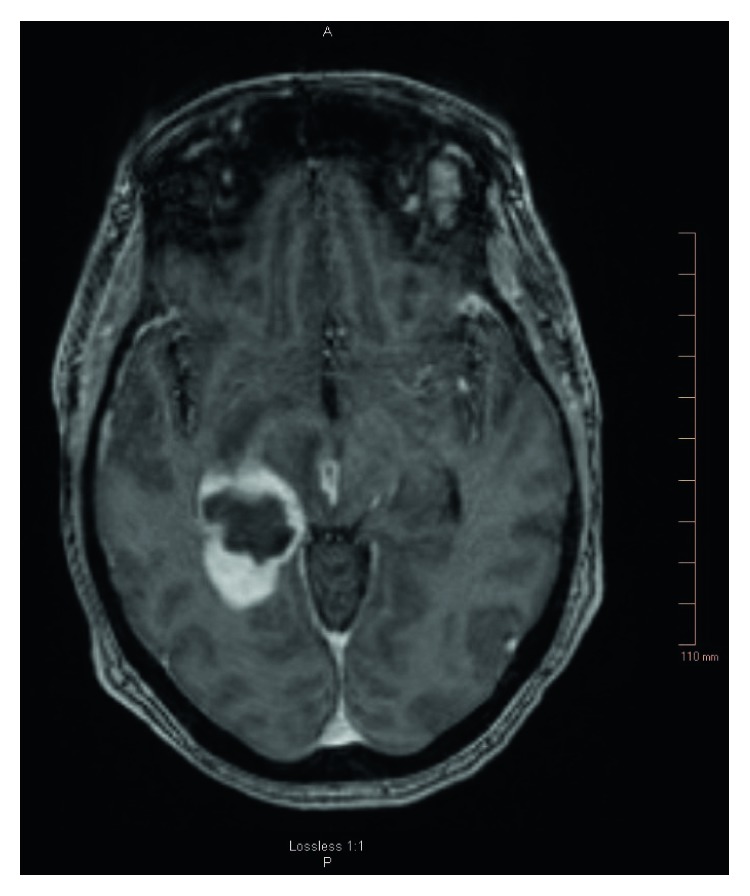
MRI brain T1 MPR TA reveals right thalamic and right medial-temporal/occipital parenchymal abscesses with extensive vasogenic edema.
